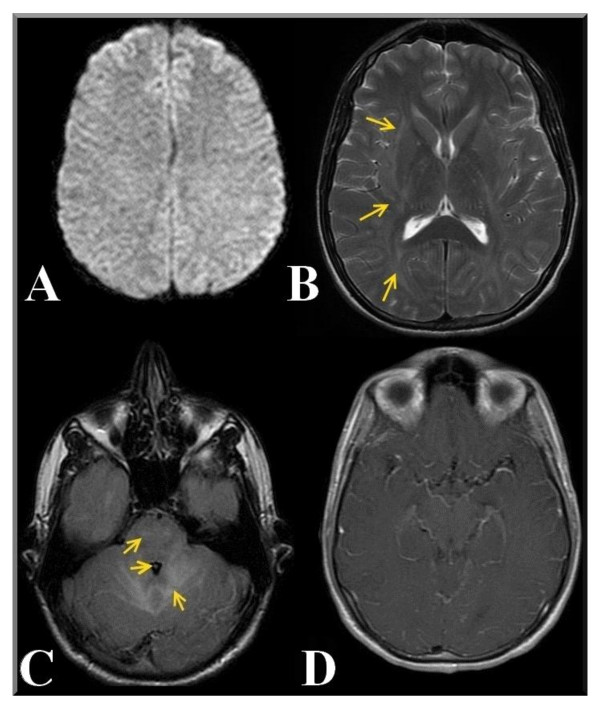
Initial MR imaging of the patient. Magnetic Resonance Imaging of the brain in the acute phase of neurological involvement. Diffusion Weighted MR Imaging of the brain performed in the acute phase of encephalitis (A). Axial T2 FRFSE image shows hyperintensity of white matter with involvement of internal and external capsule (B). Axial T2 FLAIR image demonstrates hyperintensity of white matter; involvement of the ventricular system with reduction in the size of the fourth ventricle is also shown (C). On the T1W Axial FLAIR there is enhancement of cerebral vessels after contrast agent was administered. Hyperintensity of cerebral vessel (D).
